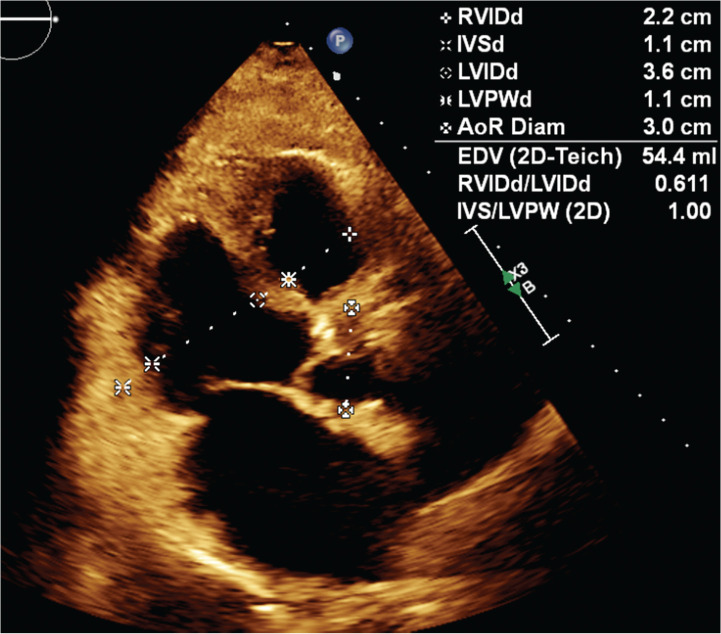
Preoperative transthoracic echocardiogram assessment with left and right ventricular dimensions. *Abbreviations:* AoR Diam, aortic root diameter; EDV, end-diastolic volume; IVS, interventricular septum; IVSd, interventricular septal thickness in diastole; LVIDd, left ventricular internal diameter in diastole; LVPW, left ventricular posterior wall; LVPWd, left ventricular posterior wall thickness in diastole; RVIDd, right ventricular internal diameter in diastole.
